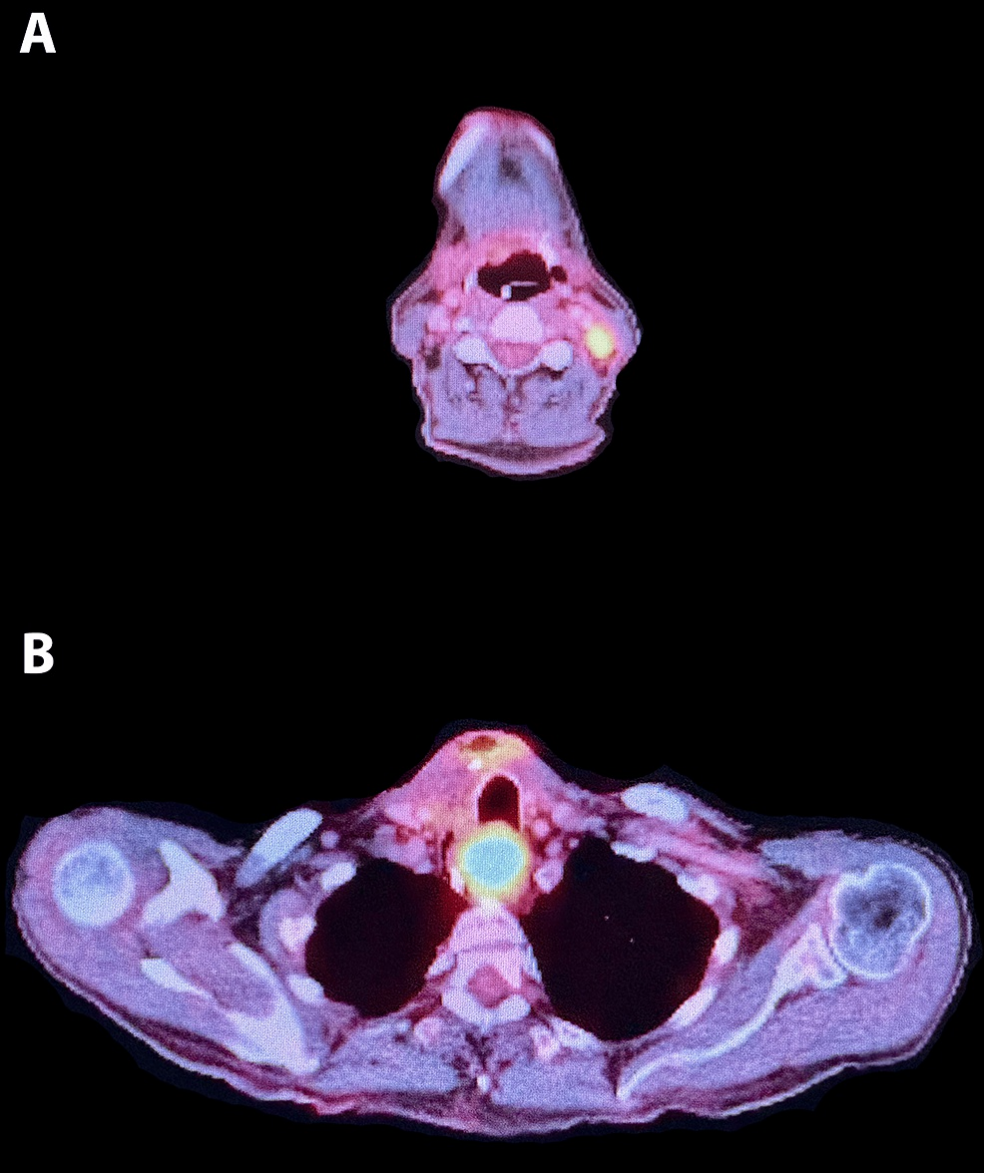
Post-operative positron emission tomography-computed tomography (PET/CT) scan. A: Axial image: hypermetabolic left level II cervical neck lymph node, highly worrisome for malignancy. B: Axial image: intensely hypermetabolic soft tissue mass in the proximal esophagus, highly worrisome for malignancy. Prominent metabolic activity in the thyroid surgical bed, favoring post-surgical inflammatory changes. Metabolically active right level II and IV cervical neck lymph nodes, the relatively mild diffuse nature of the activity, favoring reactive/inflammatory changes.
